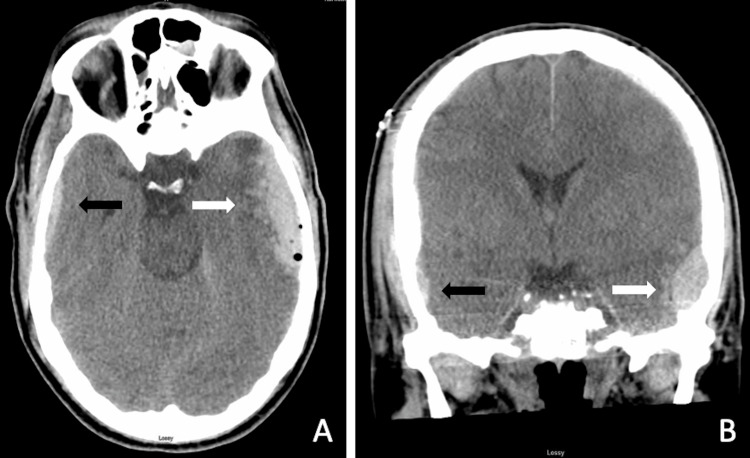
(A, B) Non-contrast CTH with left subdural hematoma and cerebral contusion (white arrow) and right epidural hematoma (black arrow). CTH, computed tomography of the head
